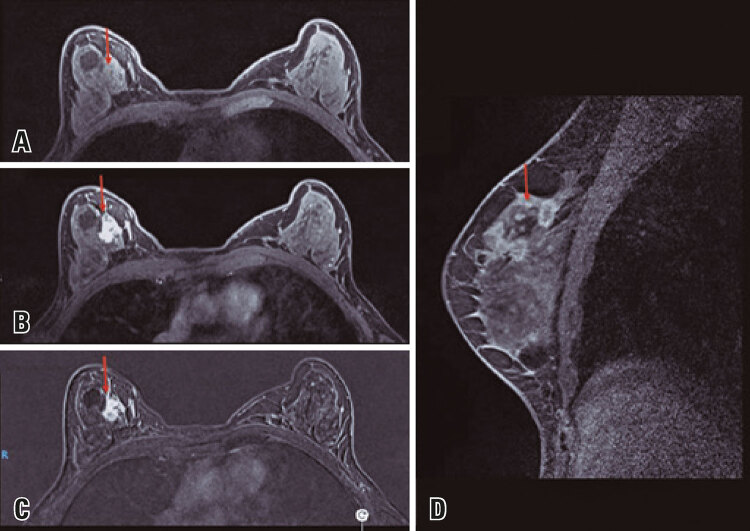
Female, 48 years old, family history of breast cancer. Magnetic resonance imaging revealed irregular and spiculated masses in the right breast, with heterogeneous enhancement and type III dynamic curve (red arrows), consistent with ultrasonographic and mammographic findings described on the same date and classified as BI-RADS 5. (A) Fat-suppressed pre-contrast T1 image; (B) Second post-contrast T1 sequence; (C) Subtraction image (post-contrast - pre-contrast); (D) Sagittal reconstruction of post-contrast image
